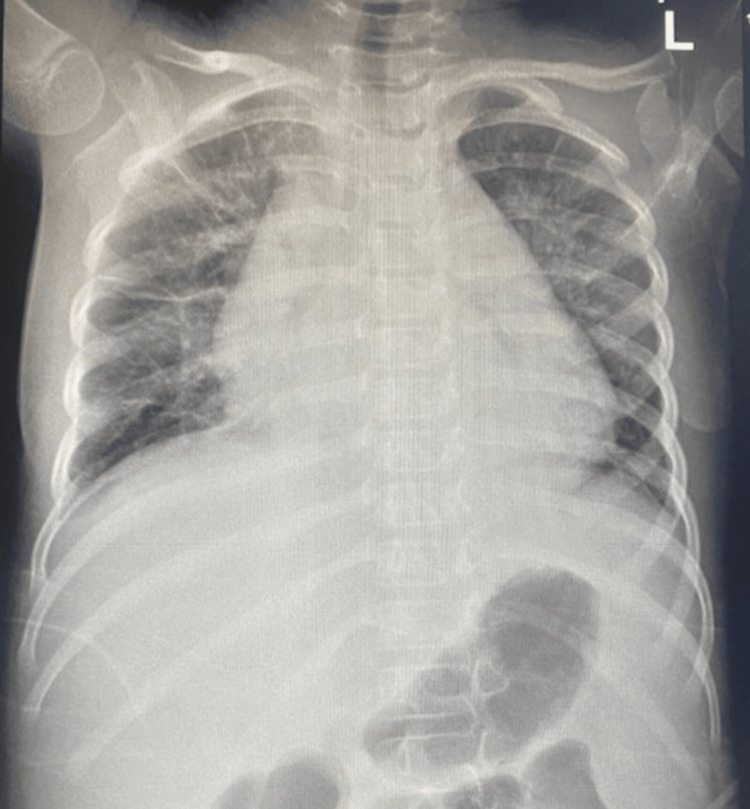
Chest X-ray day 4 of PICU admission PICU: paediatric intensive care unit
